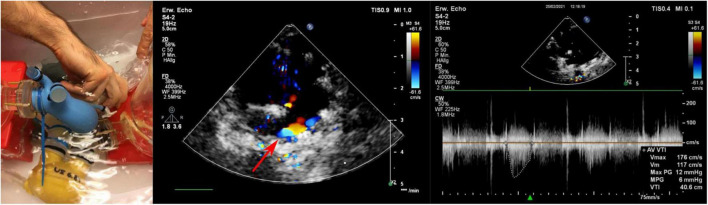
Echocardiographic acquisition (left) and determination of PVLs by Doppler (middle) and continuous wave (right) measurements.
